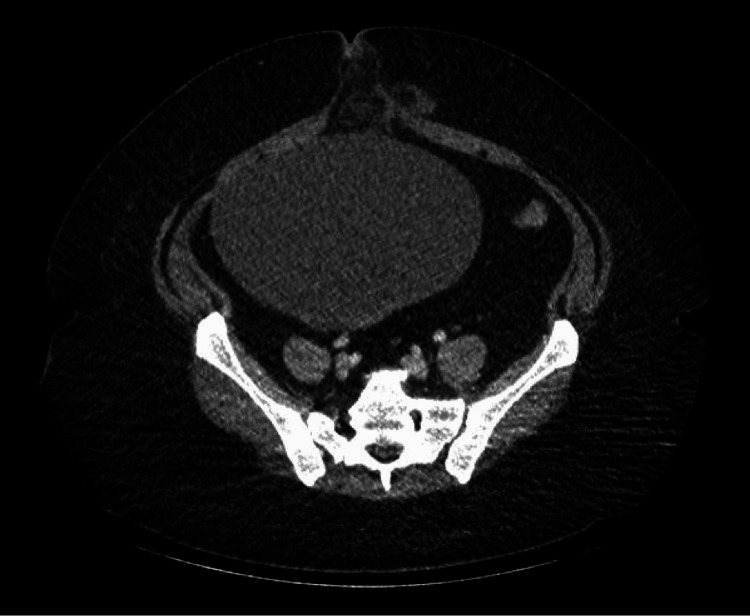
Abdominal CT scan showing a large cystic mass with an overlying fat-containing umbilical hernia
